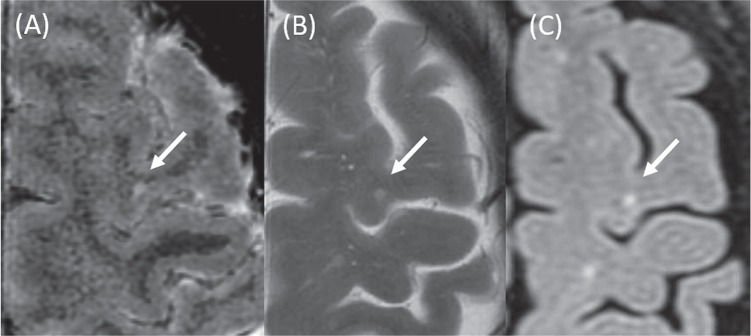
A juxtacortical multiple sclerosis lesion visualized on a PADRE (A), a T_2_-weighted imaging (B), and a 3D FLAIR image (C). The PADRE image shows a lesion involving both the gray matter and U-fiber. On the other images, involvement of the U-fiber only was recognized (arrows). 3D FLAIR, three-dimensional fluid-attenuated inversion recovery; PADRE, phase difference enhanced imaging.
